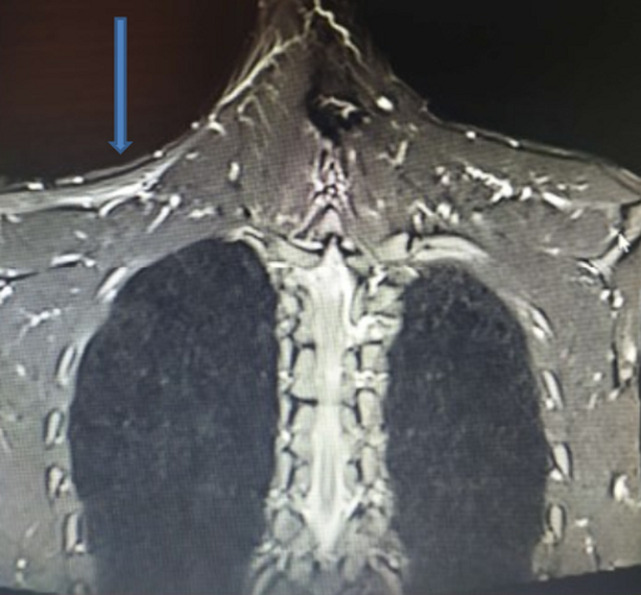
MRI of the brachial plexus, coronal cut in T2 sequence: wasting of the right trapezius muscle with denervation edema
